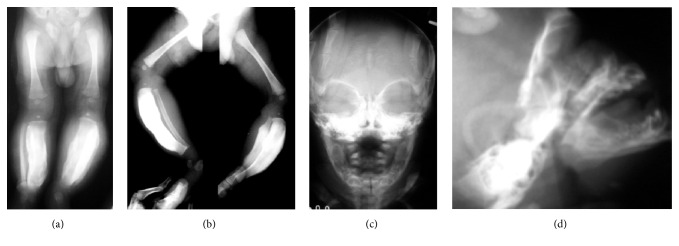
Radiographs of the cortical hyperostosis. ((a) and (b)) Massive cortical thickening and bowing of both tibias are shown. Thickening of left fibula is observed. Soft parts of both legs are thickened in frontal and lateral view. ((c) and (d)) Massive external cortical of mandible in frontal and lateral projection. Mandible is swollen.
